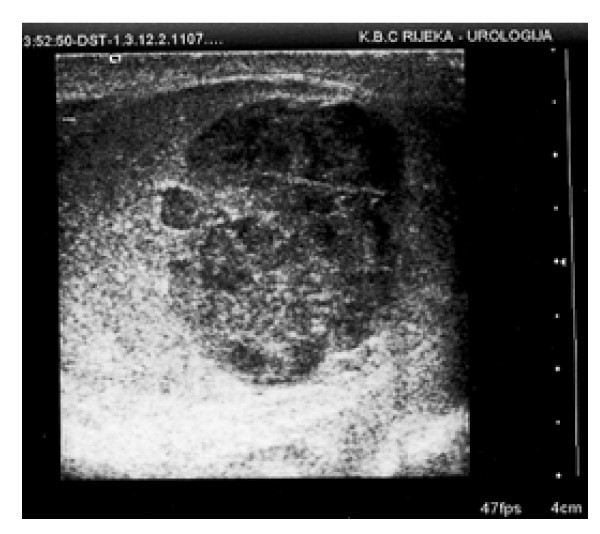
Ultrasound: hypoechogenic focal lesion in the lower pole of right testicle.
